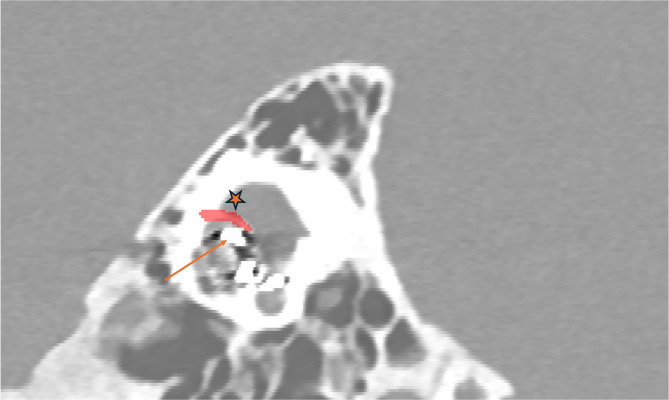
Coronal PC-CT image of the temporal bone in a non-FAO patient without FNS, showing manual bone segmentation (in red) between the middle electrode of the cochlear implant and the facial nerve using ITK-SNAP. The orange arrow indicates the cochlear implant’s middle electrodes, and the orange star marks the facial nerve.
